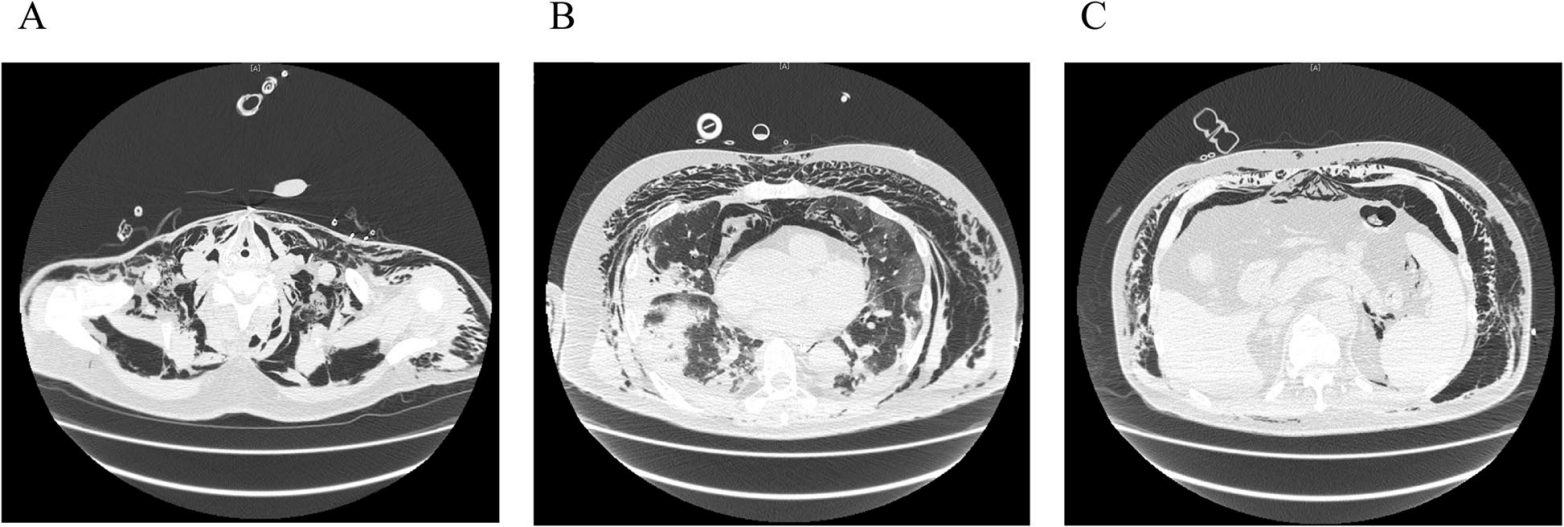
Chest CT on admission. A At the level of the neck. B Lung bases. C At the level of the pancreas. These images show bilateral diffuse ground-glass opacity with extensive basal consolidation and extensive pneumomediastinum/subcutaneous emphysema from the neck to abdomen
